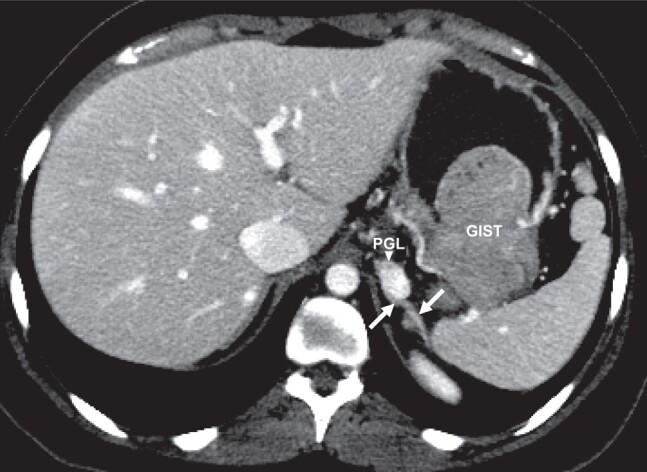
Incomplete Carney Triad in a 20-year-old woman presenting with hematemesis and diagnosed with metastatic gastrointestinal stromal tumor (GIST). An axial image from a contrast-enhanced computed tomography of the abdomen shows a GIST and a paraganglioma (PGL) located adjacent to the normal left adrenal gland (arrows).
